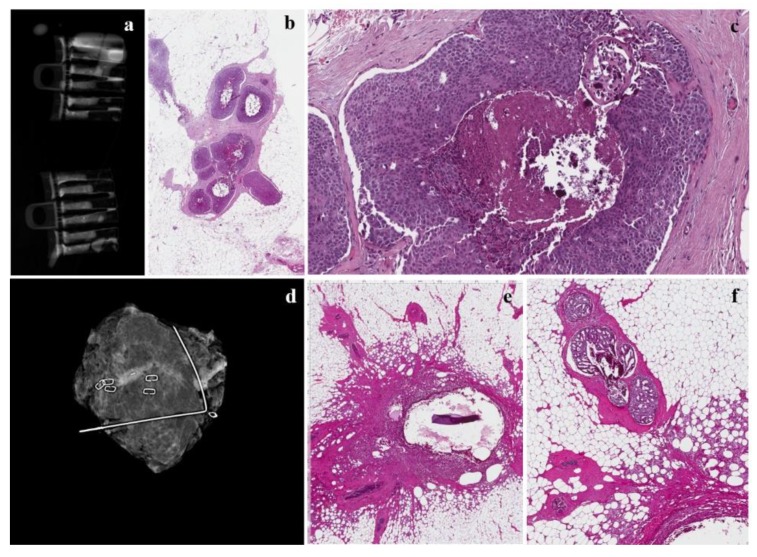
The same case as Figure 3. (a) Radiograph during localization of the microcalcification in the samples collected in touch-free collection chambers using the Mammotome Revolve 10 gauge biopsy system that reveals numerous microcalcifications in the cores; (b,c) mammotome biopsy shows a high-grade DCIS with central comedonecrosis at low- and high-magnification; (d) mammogram revealing successful retrieval of a cluster of pleomorphic calcifications with prior localization and subsequent surgery; (e) inflammatory reaction around the previously placed clips; (f) a residual focus of cribriform carcinoma in situwith central microcalcifications.
